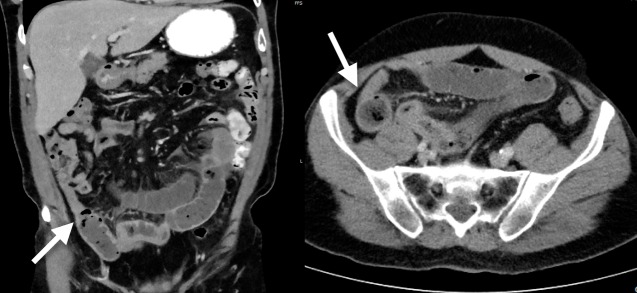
CT scan of the abdomen showing SBO and transition point (white arrow) CT: computed tomography; SBO: small bowel obstruction.
